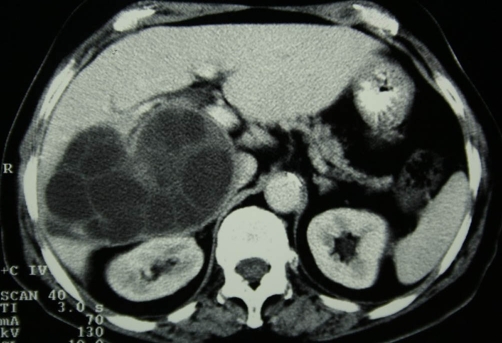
Contrast enhanced CT section at the level of the right subhepatic space. A large multilobular cystic mass of the right liver lobe is present extending to the subhepatic space.
